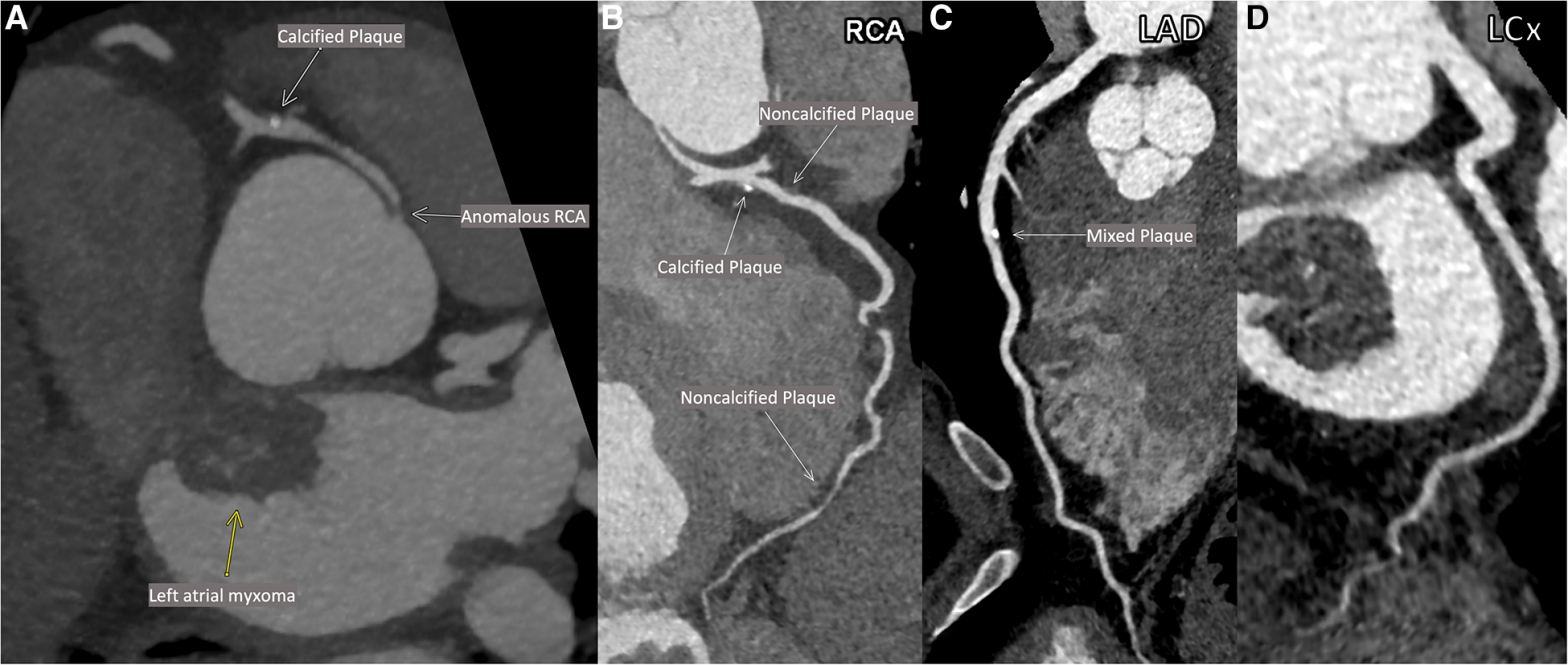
Patient's coronary computed tomographic angiography (CCTA). Anomalous origin of the right coronary artery (RCA; A). Multiplanar reconstructions of the RCA (B), the left anterior descending artery (LAD; C) and the left circumflex artery (LCx; D).
